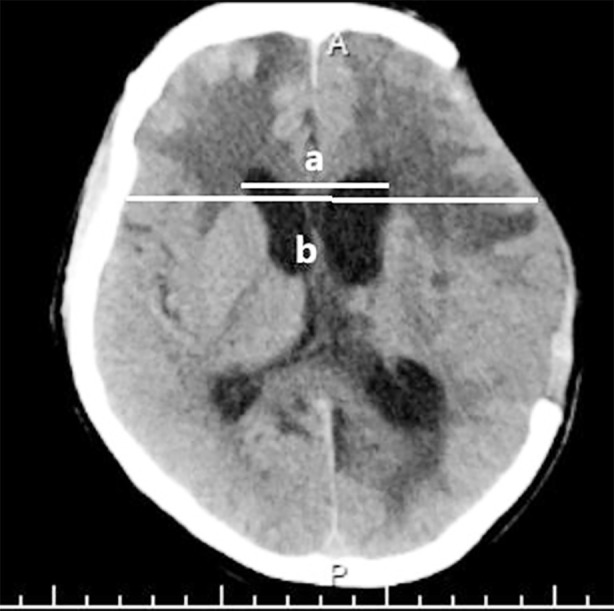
Axial cranial CT scan after left routine frontal-temporal-parietal decompressive craniectomy to measure the modified frontal angle (a/b).
